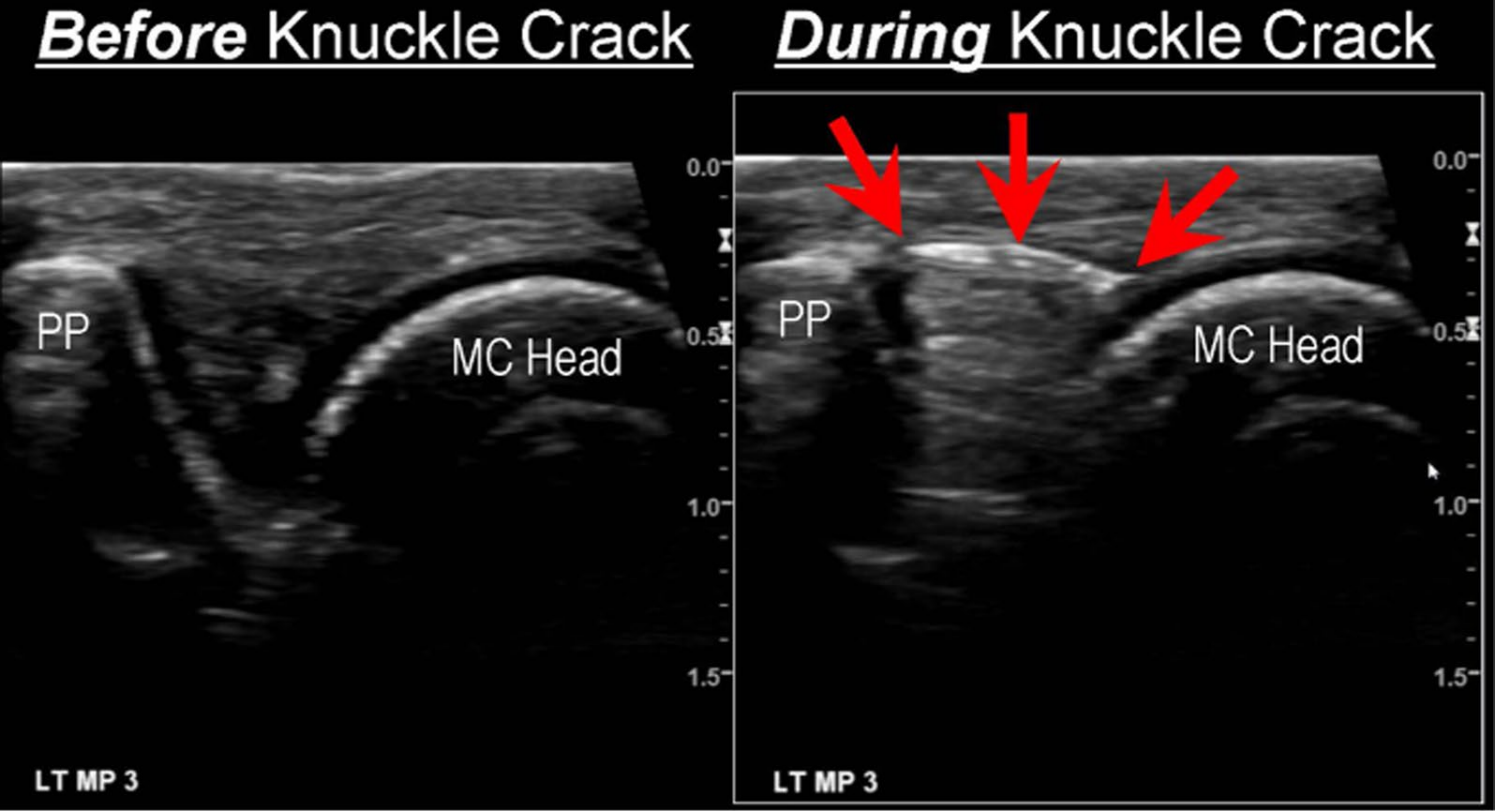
Ultrasound images before and after cavitation in an MCP joint. From Boutin et al. [34]
